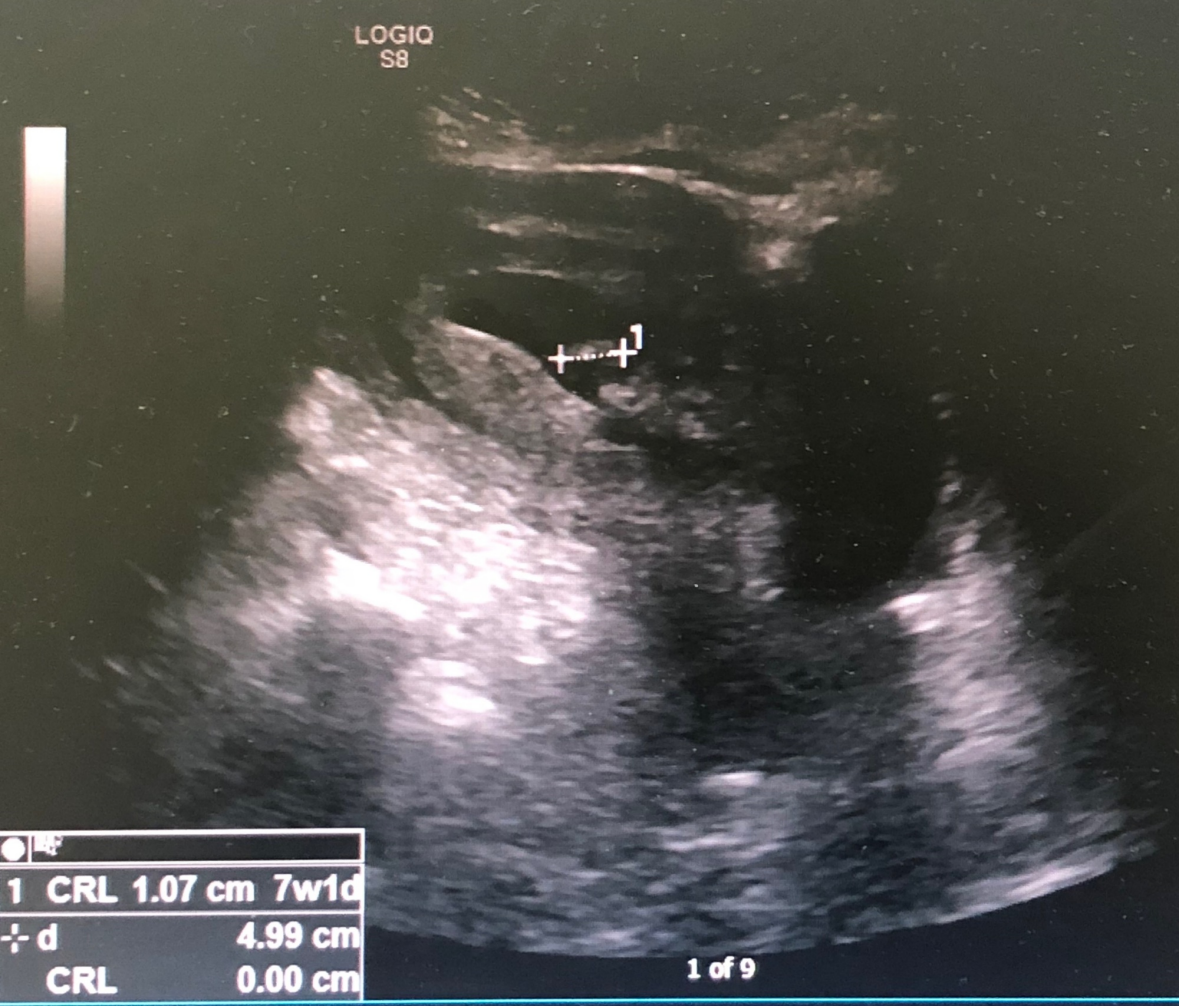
Ultrasound showing an intrauterine single nonviable fetus with a crown-rump length corresponding to seven weeks
